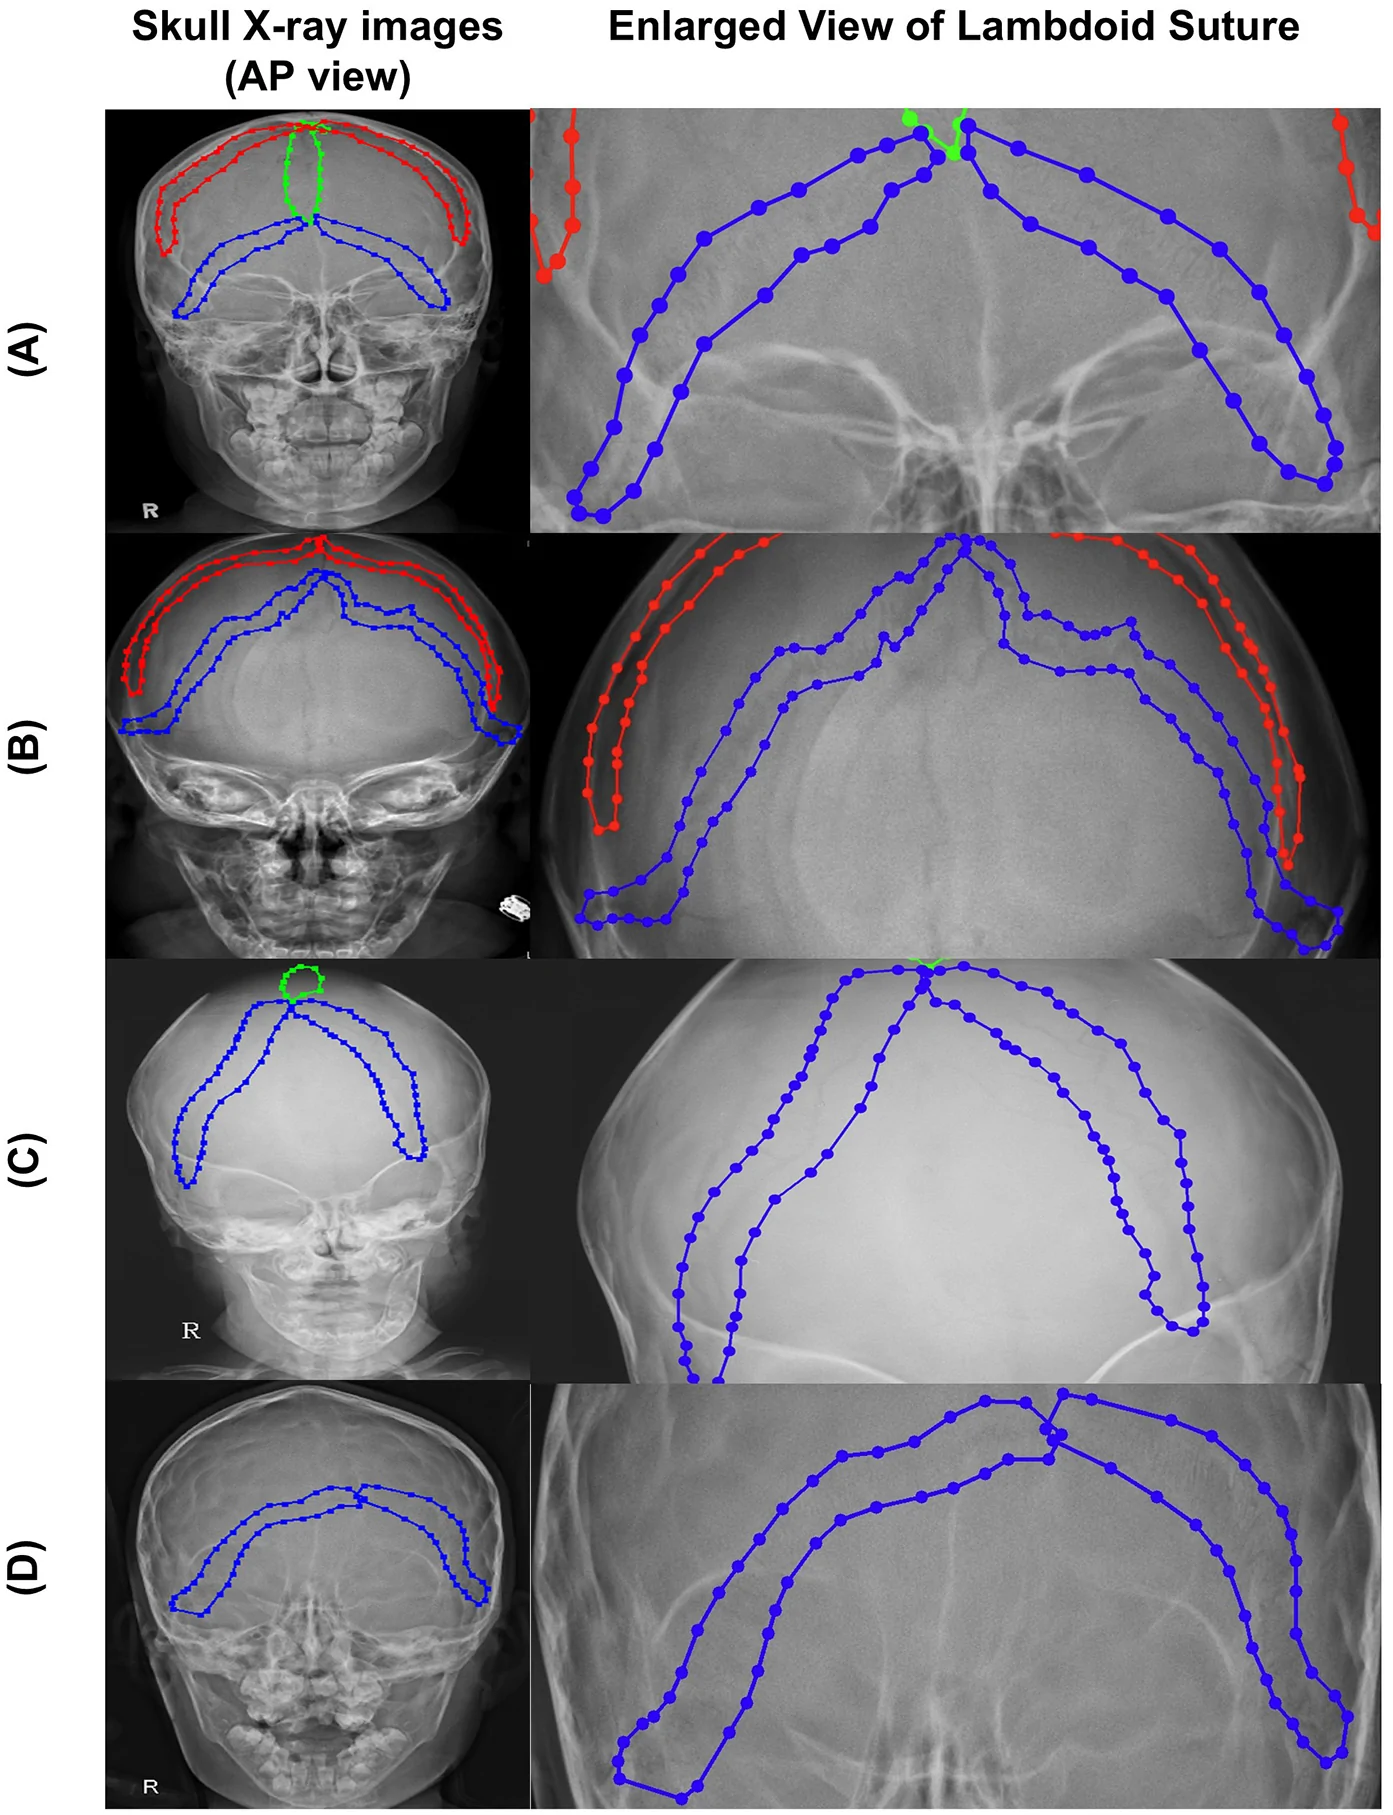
Skull X-ray images (AP view) and enlarged views of the lambdoid suture for four diagnostic types. (A) Normal. (B) Sagittal. (C) Coronal(Bi). (D) Coronal(Bi) and Sagittal.
